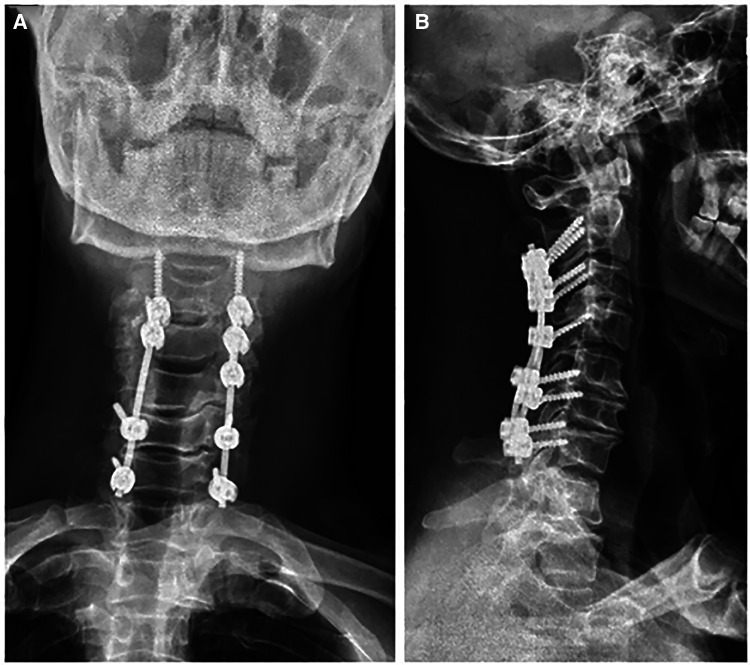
Postoperative x-ray [(A) anterio-posterially and (B) laterally] showed a satisfactory posterior fixation of cervical spine from C2–7.
